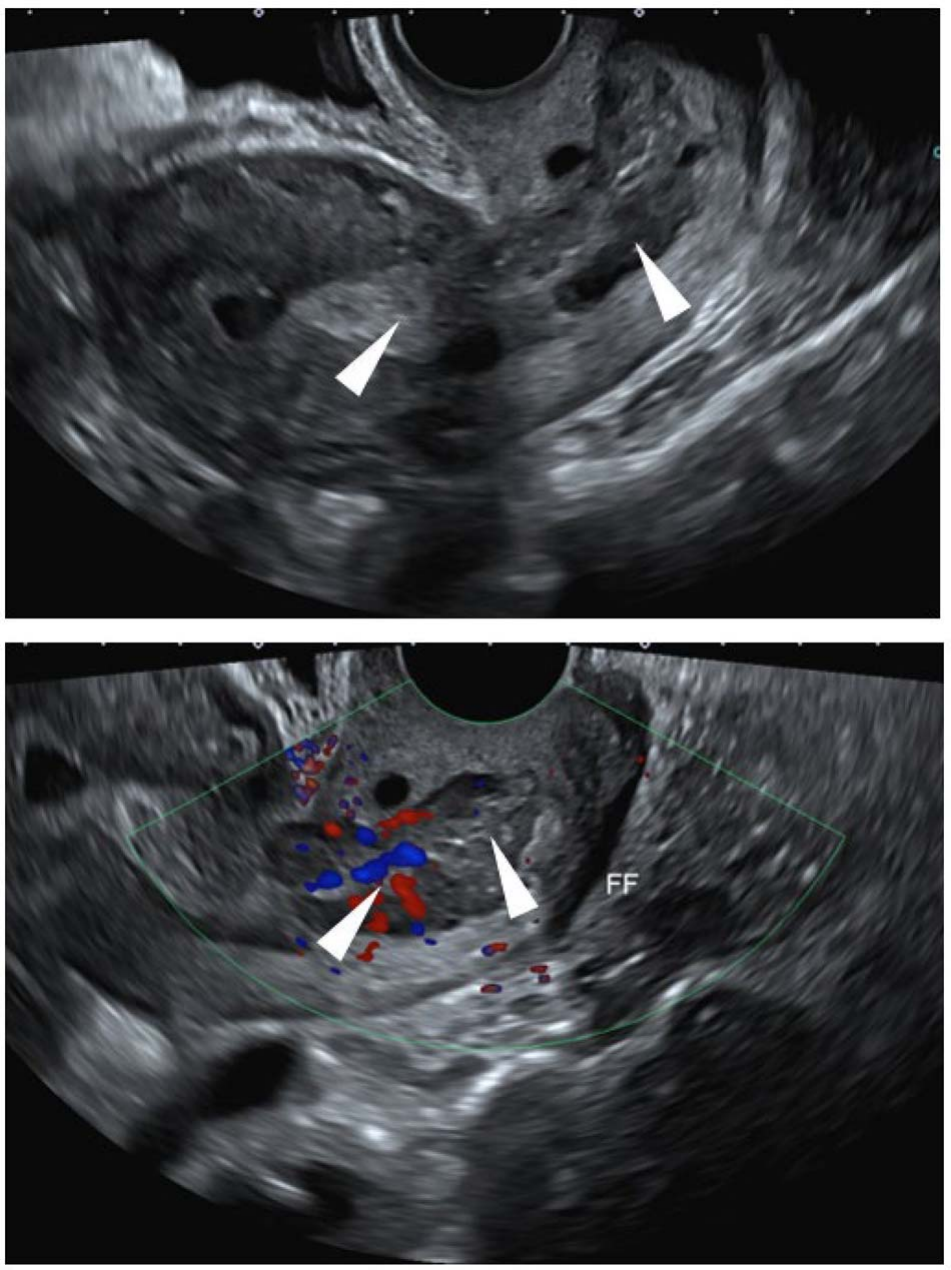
Ultrasound: Transvaginal pelvic ultrasound demonstrates thickening and heterogeneity in the lower endometrial stripe and endocervical canal (arrowheads). There is also pelvic free fluid (FF).
